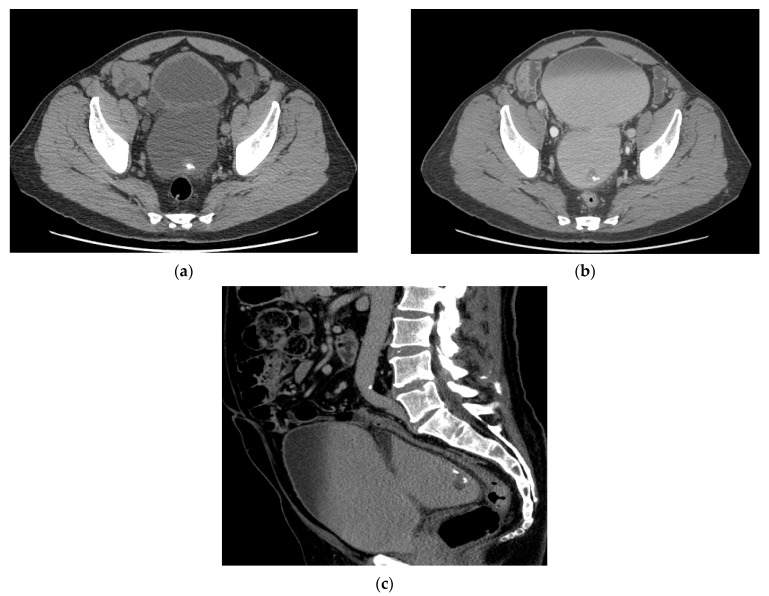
CT urogram with partially calcified urothelial carcinoma within a large bladder diverticulum (a) Axial CT image obtained without contrast shows a large bladder diverticulum containing a partially calcified lesion along the posterior aspect representing urothelial carcinoma. (b) Axial CT image obtained during the excretory phase and (c) Sagittal excretory phase image show the same mass as a filling defect surrounded by excreted urine in the bladder.
